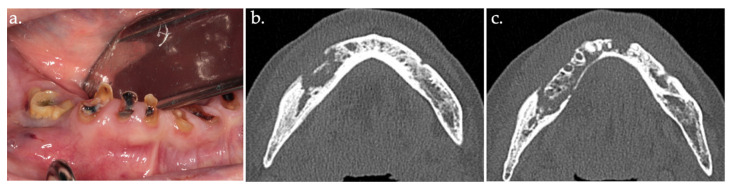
Intraoral view showing stage 2 MRONJ, with exposed sequestrum (a); CT scan image (b,c).
